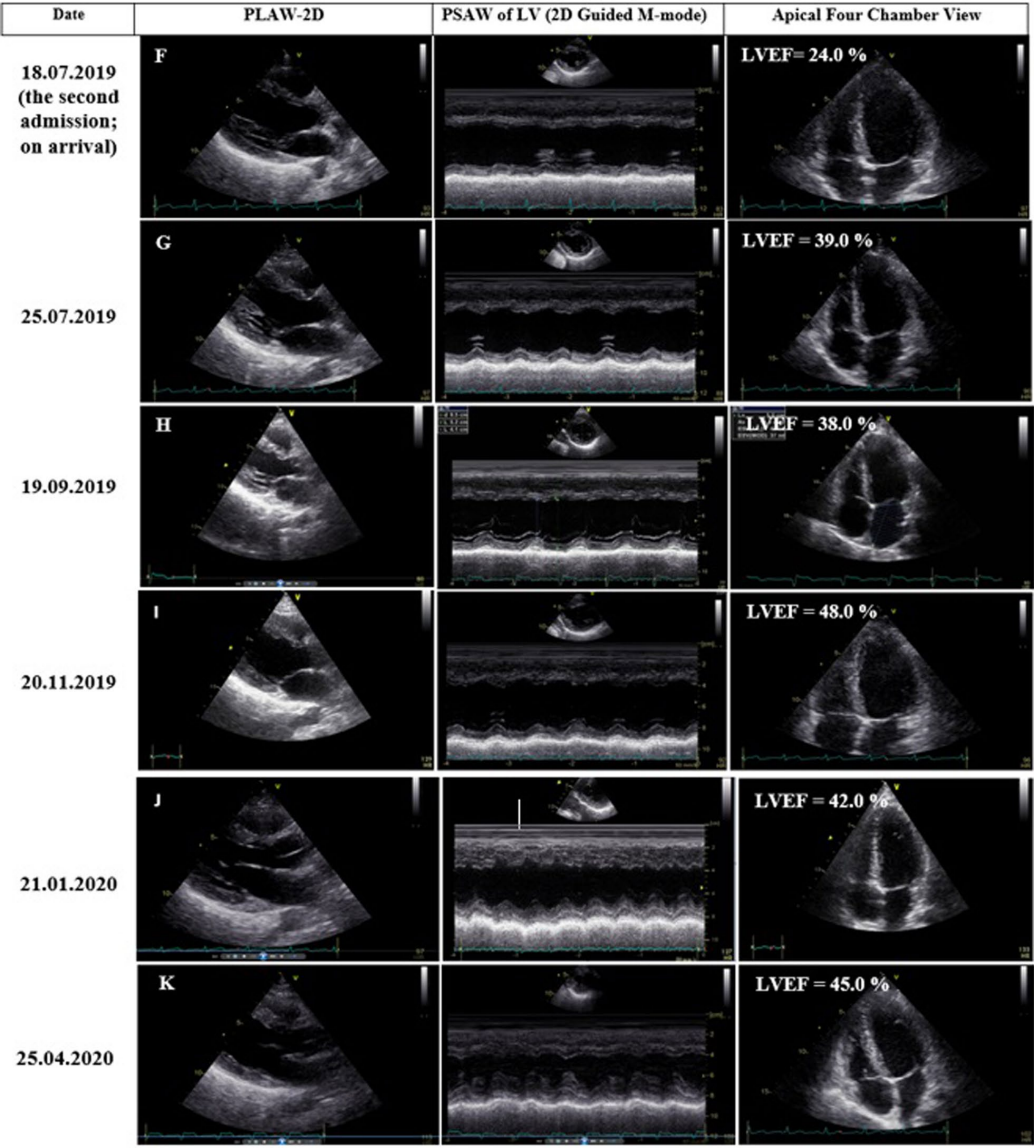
Serial transthoracic echocardiographic studies. A–E views: first admission and later follow-ups. On arrival, there was a large amount of pericardial effusion in addition to left ventricular systolic dysfunction. During the hospital course and then after, there were improvements in the amount of pericardial effusion, as well as thickness and systolic function of the myocardium. F–K views: second admission and later follow-ups. On arrival, there was systolic dysfunction in addition to scanty thinning of the ventricular myocardium. Later in and out of the hospital, there were improvements in the myocardial thickness, as well as the systolic function of the myocardium
